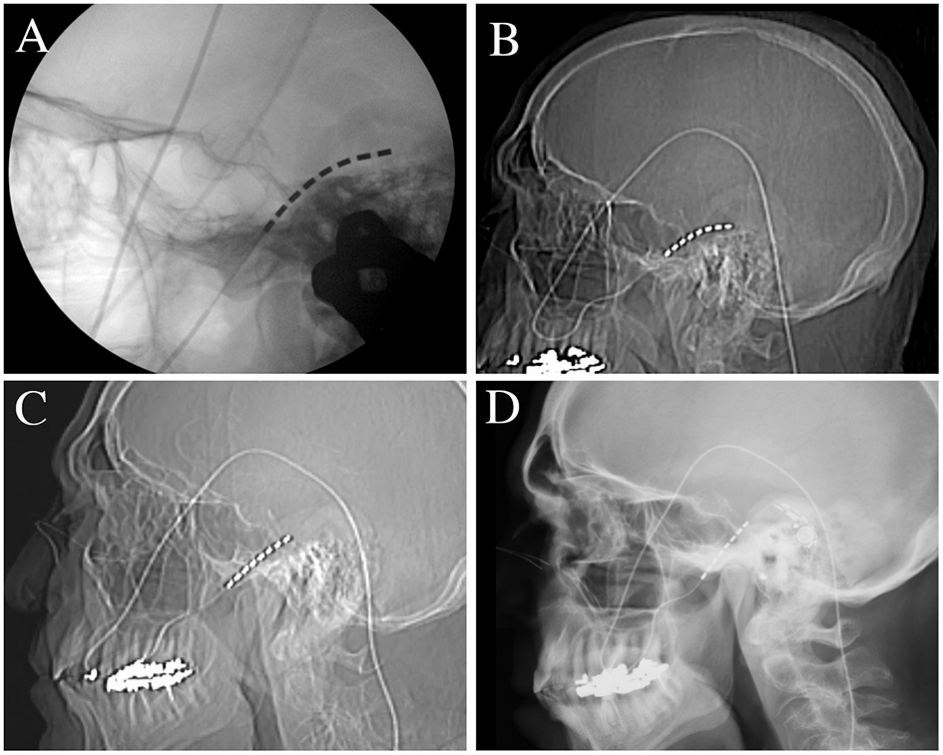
Radiographs of permanent electrode placement, secured with the novel anchoring technique. (A) Intra-operative lateral skull radiograph showing placement of the lead within the foramen ovale. (B) Immediate postoperative lateral skull radiograph showing preserved lead position. (C) Lateral skull radiograph was taken 3-days after permanent electrode implantation showing mild descent of the electrode by 3 contacts (~13 mm). (D) Lateral skull radiograph performed at 6 months showing no further movement of the lead.
